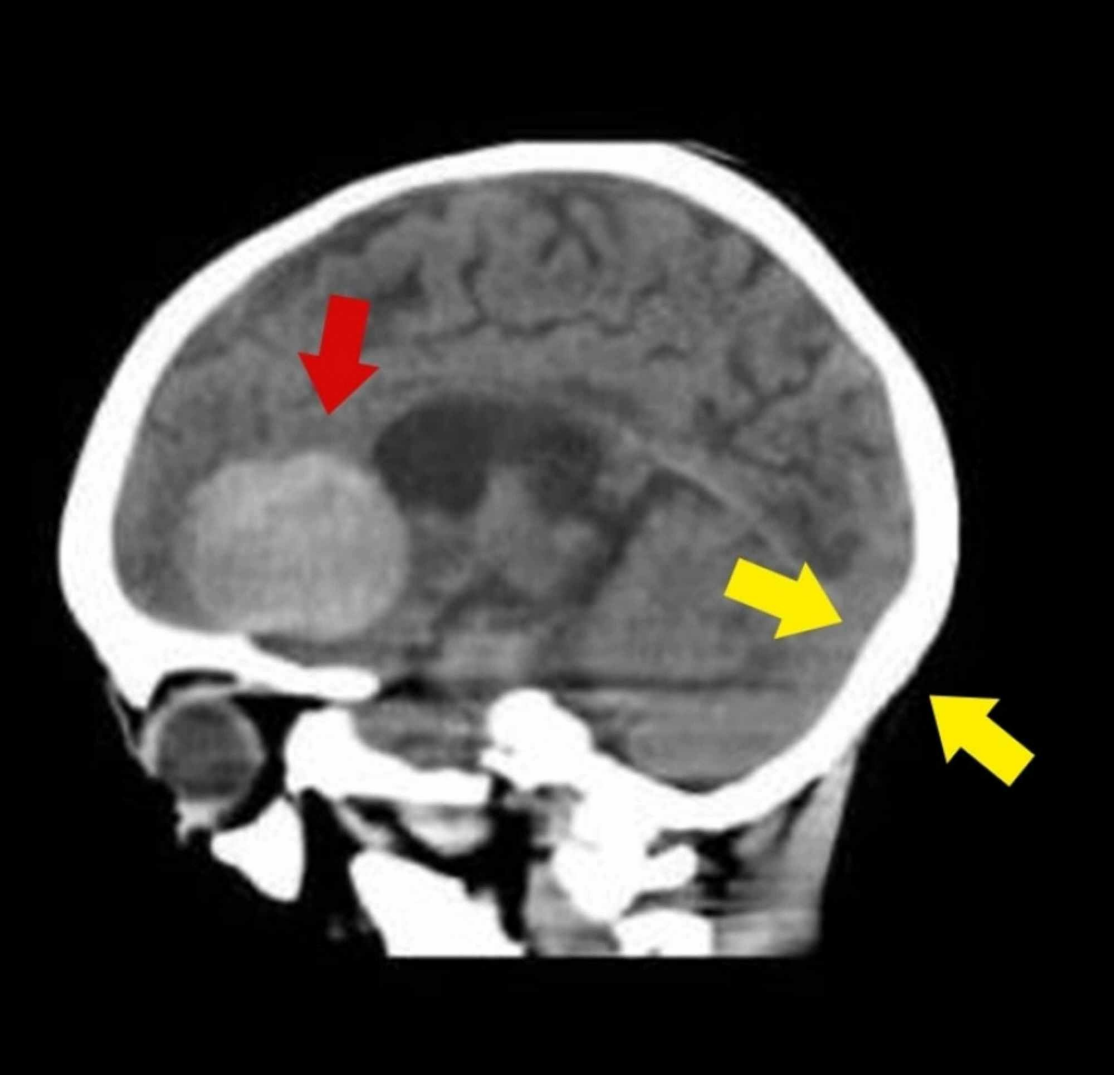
CT scan brain showing a large right frontal lobe hemorrhage (red arrow) and cortical thickening of the skull (yellow arrows).
